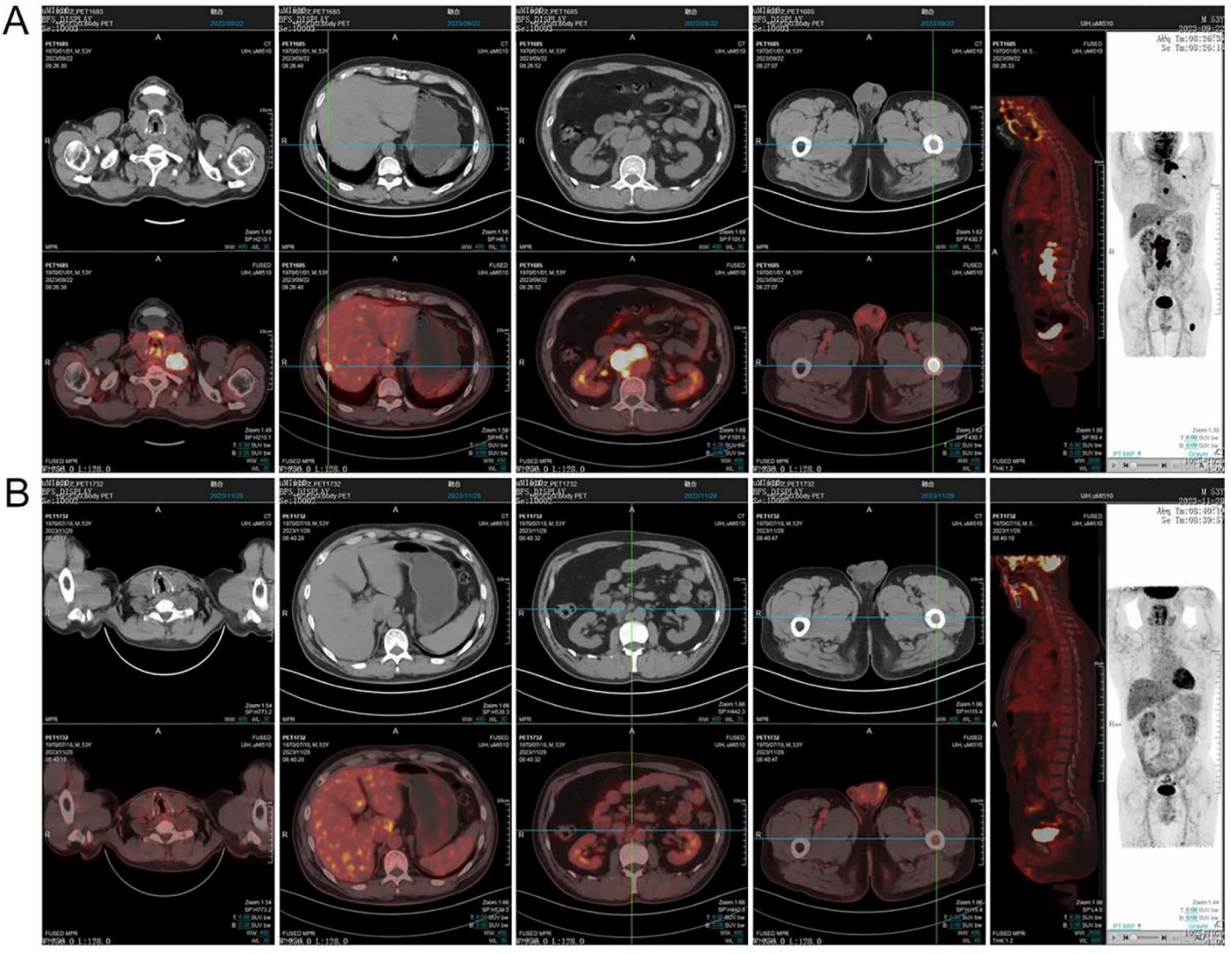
PET-CT imaging was performed at the time of initial diagnosis (A) and after two cycles of chemotherapy (B).
